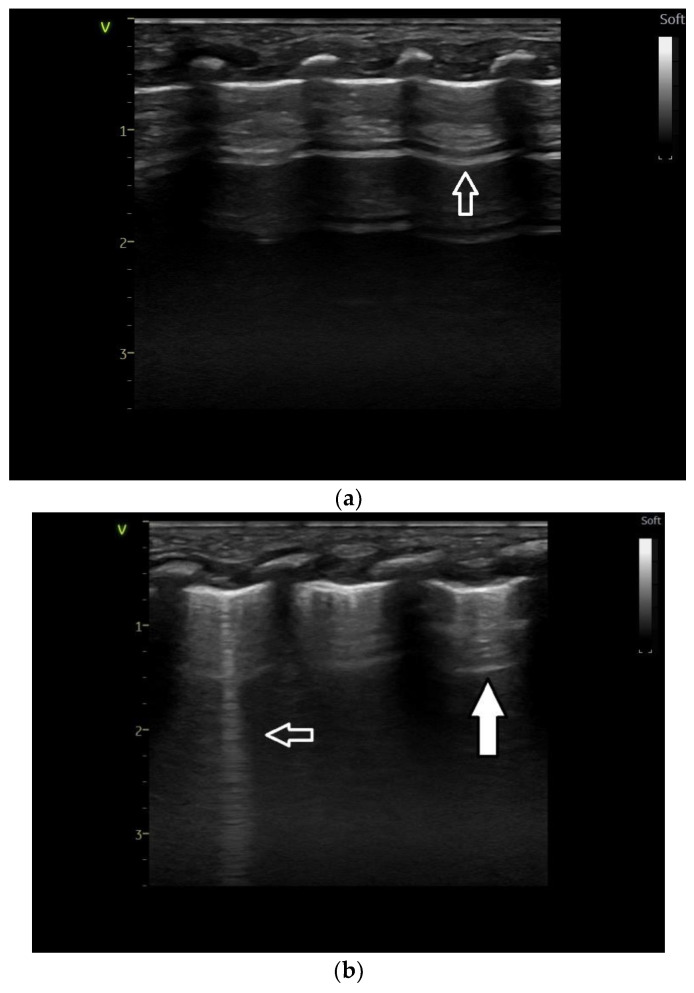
(a) Lung ultrasound. Normal image: A line—arrow—horizontal line parallel to the pleural line; (b) lung ultrasound. Normal image: A line—horizontal line parallel to the pleural line—reflection of the parietal pleura—white arrow—B line—white contour arrow—vertical line, with the origin at the visceral pleura, like a laser beam.
